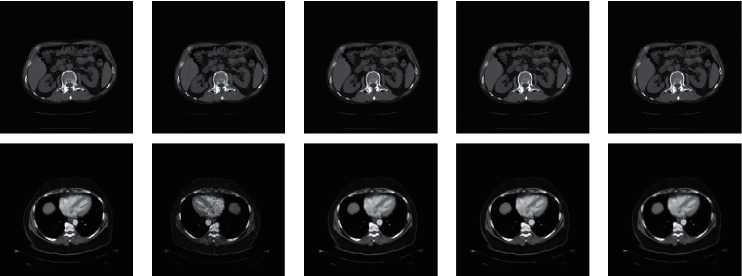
Denoising results comparison images. (a) Original CT image. (b) Low-dose CT image. (c) WGAN. (d) RED-CNN. (e) Proposed algorithm.
